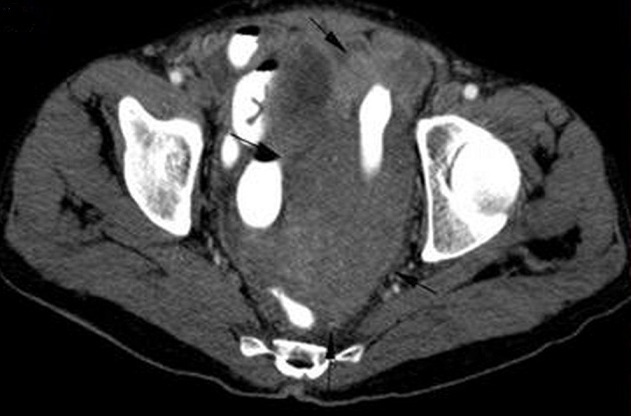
Abdominal computed tomography showing a uniform-density mass lesion of 70 mm that filled retrovesical and left pelvic area also multiple lymph nodes in the retro-vesical, celiac, para-aortic and para-iliac areas
